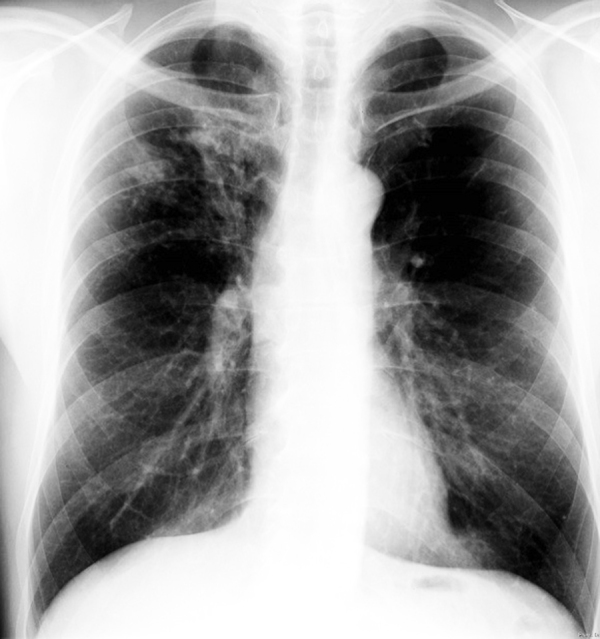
Chest x-ray in August 2010.
